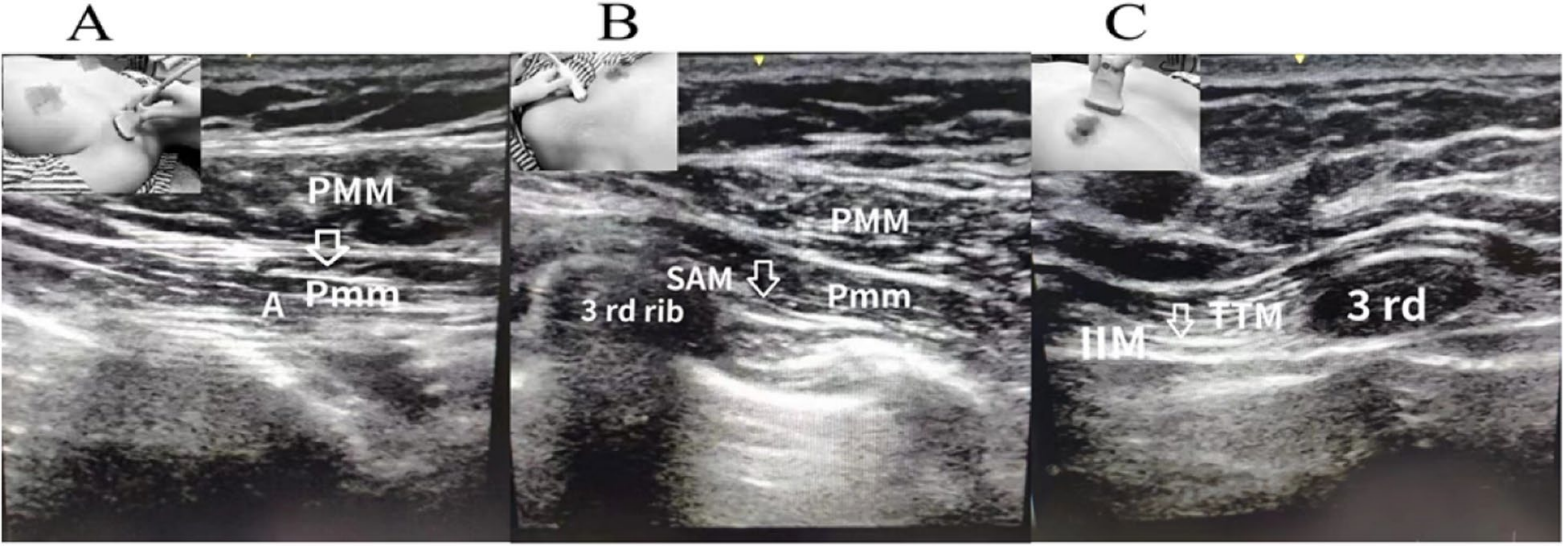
For the TTP-PECS blocks, the position of the ultrasound transducer is shown in the upper left of the images. During ultrasound scanning of PECS I block, a local anesthetic was injected in the plane between the PMM and Pmm (A); in PECS II block, a local anesthetic was injected in the plane between the Pmm and SM (B); and in TTP block, a local anesthetic was injected in the plane between the IIM and TTM (C). The arrow indicates the injection point. A, artery; PMM, pectoralis major muscle; Pmm, pectoralis minor muscle; SAM, serratus anterior muscle; IIM, internal intercostal muscle; TTM, transversus thoracic muscle
